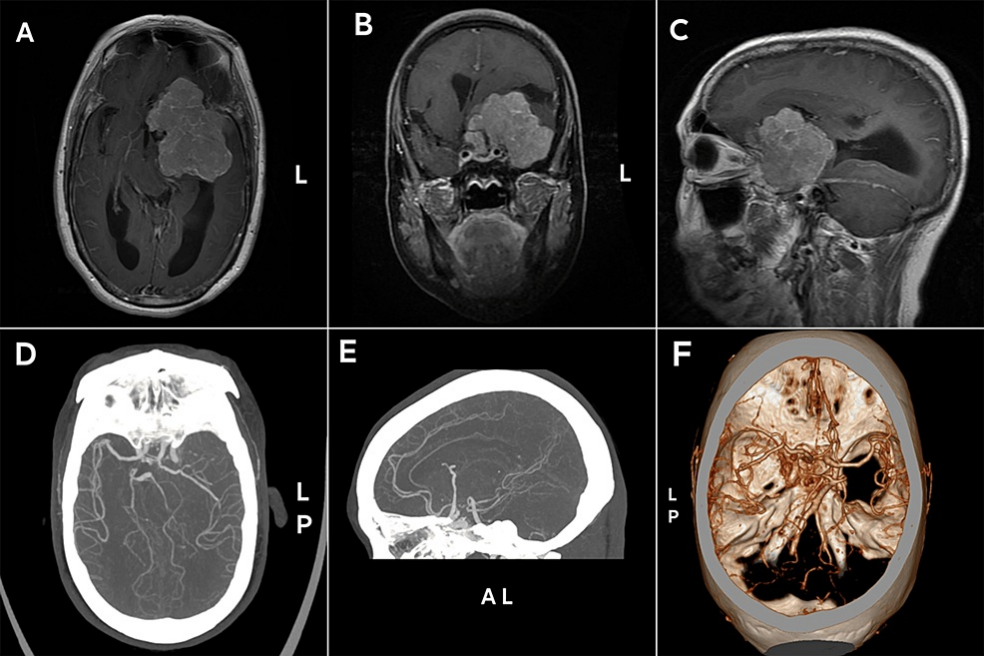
Preoperative images A: contrast-enhanced T1-weighted axial view; B: contrast-enhanced T1-weighted coronal view; C: contrast-enhanced T1-weighted sagittal view; D, E, F: computed tomography angiography coronal and sagittal views, and 3-dimensional reconstruction
